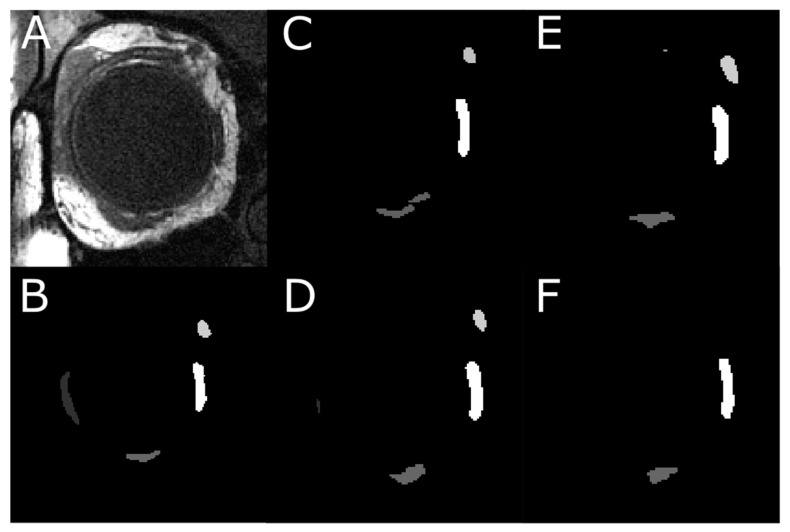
An instance of segmentation where the models do not perfectly segment the regions of interest. (A) An MR image located anteriorly in the orbit. (B) Digitally traced four EOMs. (C) Result from U-Net model, which identified SO and MR, did not manage to segment LR, and mislabeled the inferior oblique muscle as IR. (D) Result from U-NeXt model, which under-segmented LR. (E) Result from DeepLabV3+ model, which also missed LR. (F) Result from ConResNet model, which was only able to segment MR and IR.
